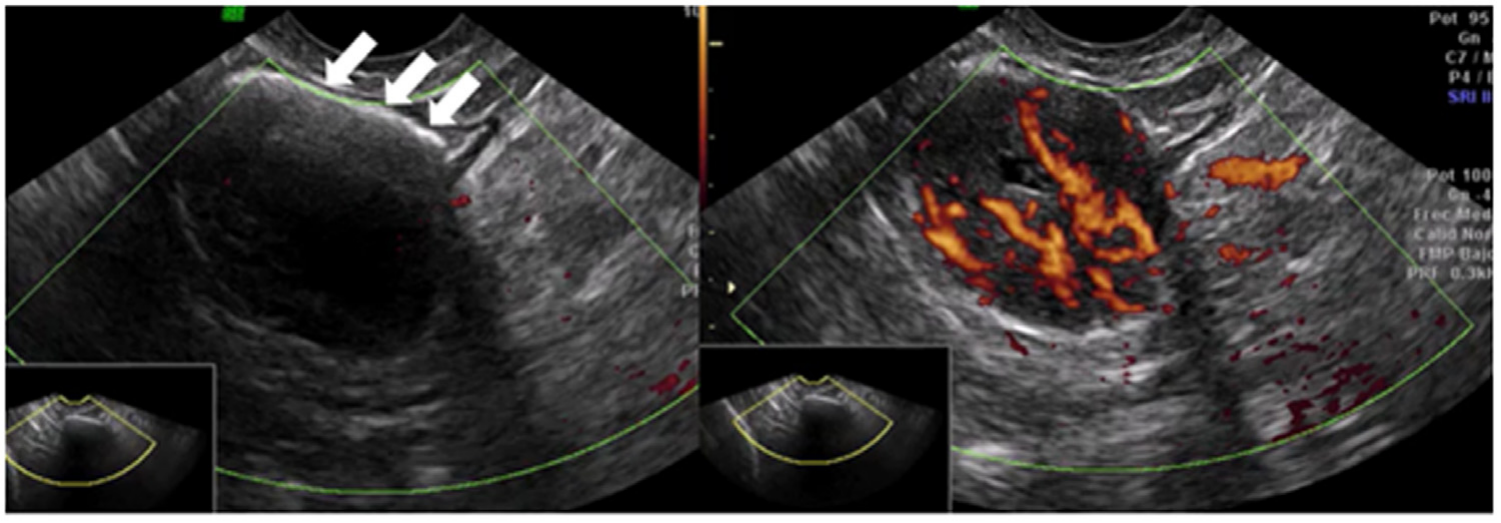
Intestinal schwannoma: transvaginal ultrasound. On the left, a hyperechogenic thin line (arrows) corresponding to intestinal gas can be seen. On the right, Power Doppler of the tumor, strongly vascularized. See also Videoclips 1 and 2.
